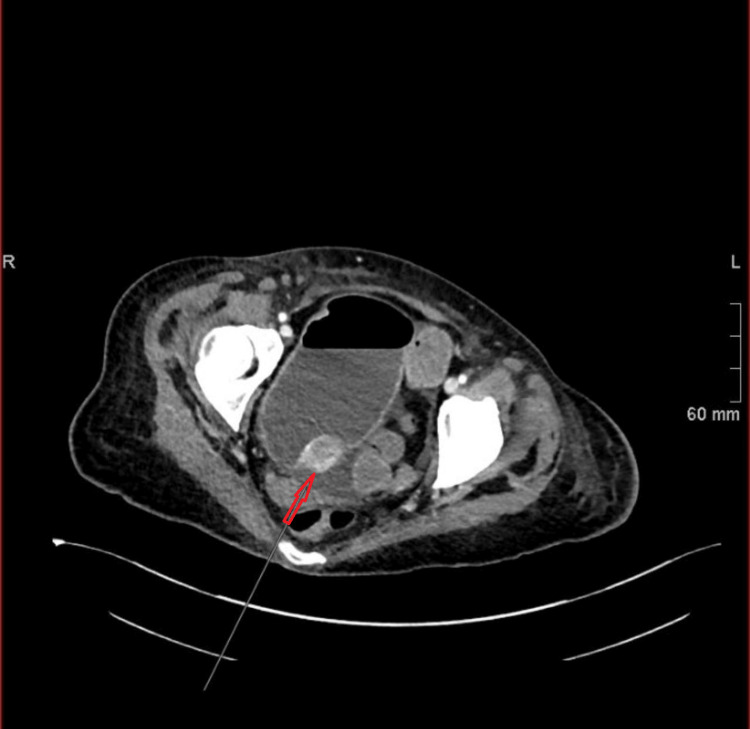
Computed tomography scan of the abdomen and pelvis demonstrating acute small bowel obstruction Axial computed tomography image of the abdomen and pelvis performed on the third day of admission following acute clinical deterioration. The image demonstrates dilated loops of small bowel with a transition point in the ileum (arrow), consistent with acute small bowel obstruction secondary to a stricturing ileal tumour. Mild ascites is also noted. CT: computed tomography.
